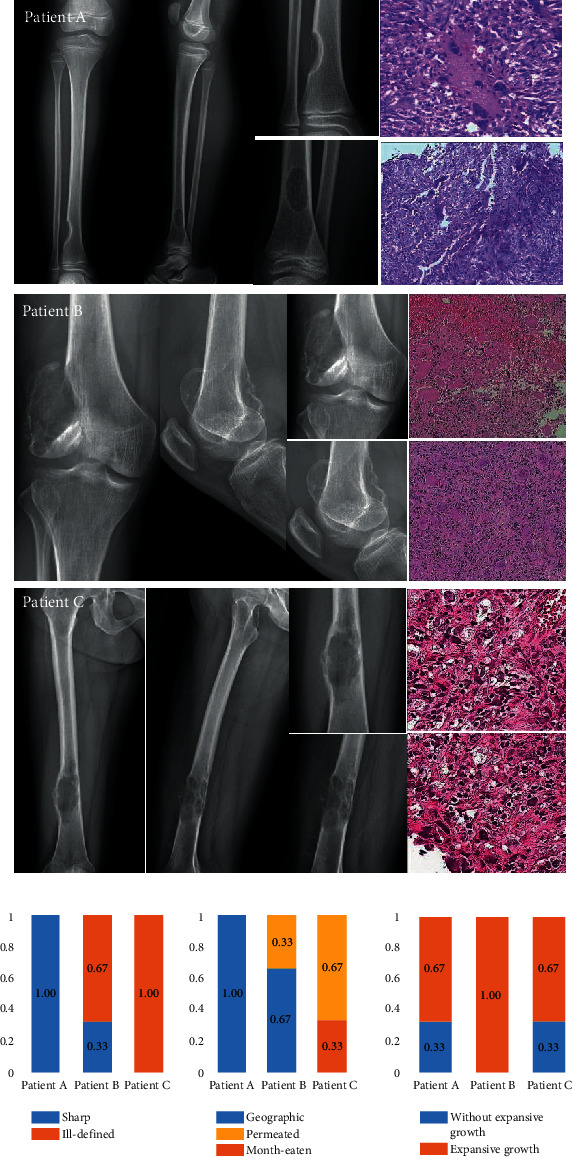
Examples of the features (upper panel) and scores depicting the presence or absence of sharp vs. ill-defined bone margins, geographic vs. moth-eaten vs. permeated pattern of bone destruction, and with vs. without expansive growth (lower panel) as seen on conventional radiographic images obtained from 3 patients. Patient A was an 8-year-old female with nonossifying fibroma. Patient B was a 34-year-old man with a giant cell tumor of bone, and Patient C was a 46-year-old woman with osteosarcoma.
